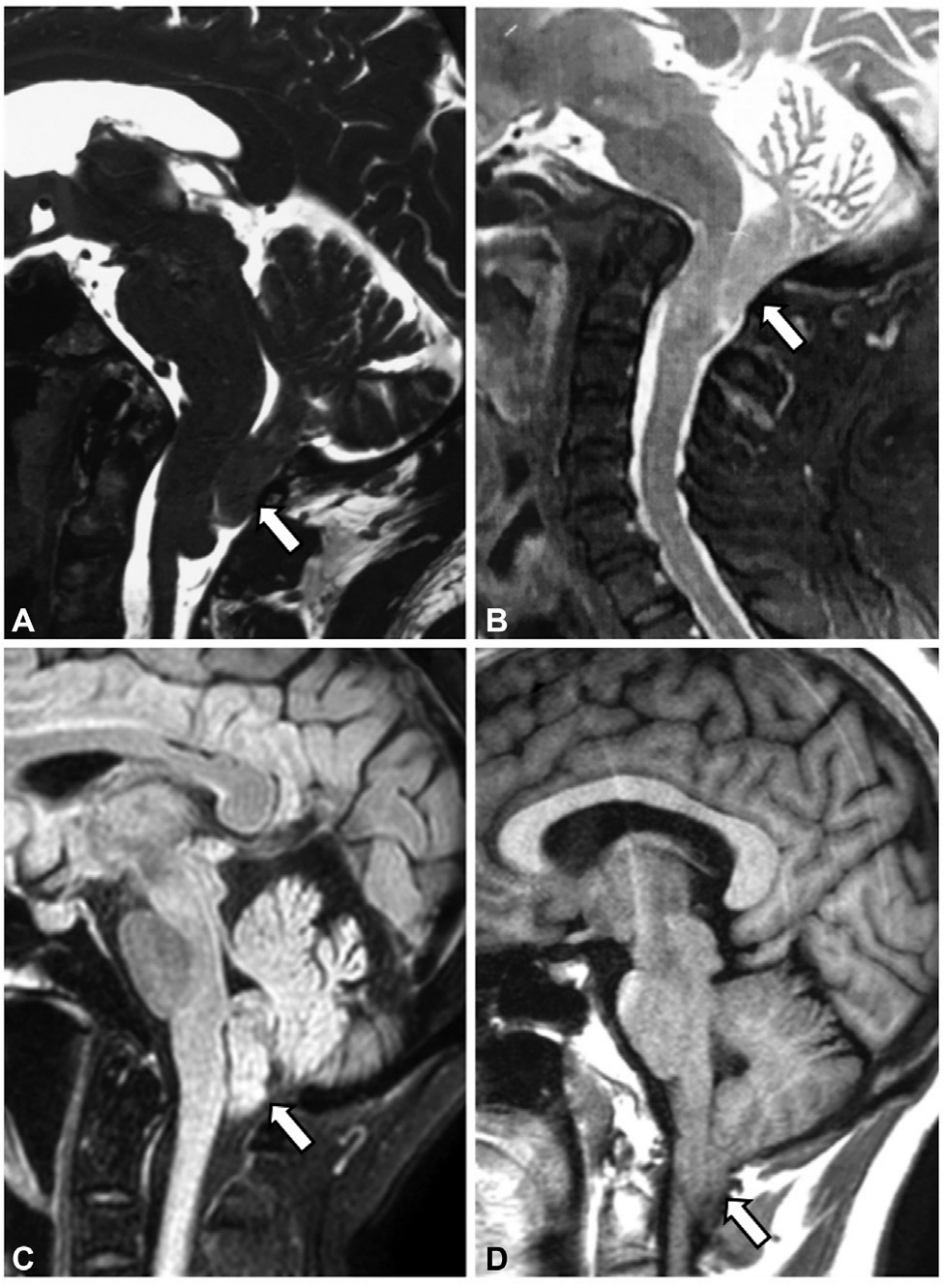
(Case A-D) Cranio-cervical MRI showing Chiari malformation type 1 in the four patients with progressive ataxia and downbeat nystagmus, which is defined by herniation of cerebellar tonsils through the foramen magnum (located at least 5 mm below this structure) (arrows).
